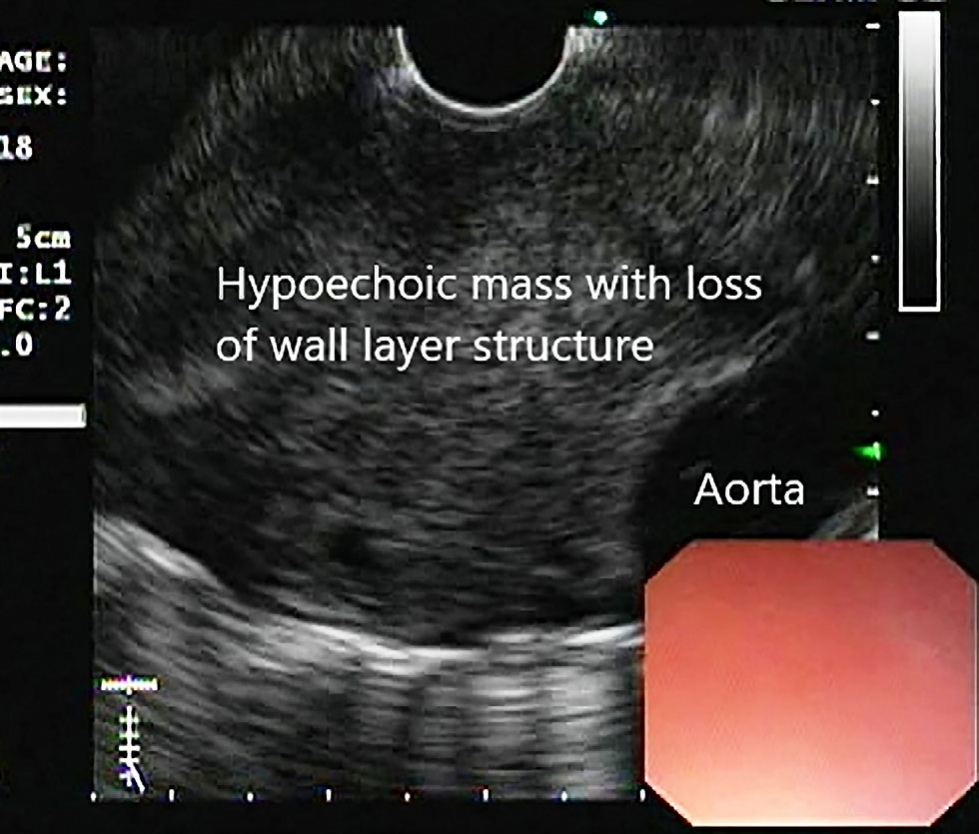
Linear endoscopic ultrasound image depicting mass with loss of wall layer structure of esophagus and mass abutting aorta.
